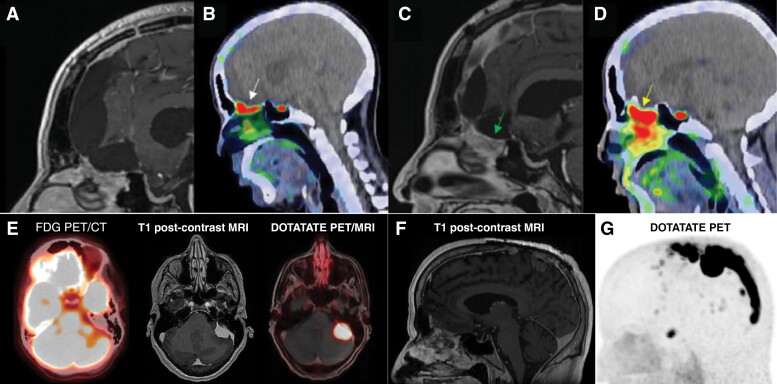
(A) Postoperative magnetic resonance imaging suggesting a gross total resection with contrast-enhancing reactive changes only. (B) Positron emission tomography (PET) imaging showing focal uptake along the cribriform plate (standardized uptake value 7.43, white arrow) suspicious for residual disease. (C) Follow-up MRI 2 years later after patient declined to pursue recommended adjuvant RT with increased enhancing soft tissue signal (arrow). (D) Increased focal PET uptake in the cribriform plate suggests progression of residual disease (standardized uptake value 8.96, yellow arrow). (E) Axial brain MRI of a different patient: a 54-year-old woman with newly diagnosed breast cancer metastatic to axillary lymph nodes who was noted to have asymmetric photopenia in the left cerebellum on a staging fluorodeoxyglucose (FDG)-position emission tomography (PET) and computer tomography (CT) scan (left). T1 post-contrast brain magnetic resonance imaging (MRI) showed a multilobulated, homogeneously enhancing extra-axial mass adjacent to the left petrous temporal bone with associated edema and mass effect in the left cerebellum and cerebellar peduncle (middle). Leading differential diagnoses included a distant metastasis or a meningioma. DOTATATE PET/MRI showed markedly avid uptake in the intracranial mass (right), but not in the right breast or ipsilateral lymph nodes (not shown). A diagnosis of synchronous meningioma and locoregionally advanced breast cancer was made. The meningioma was treated with stereotactic radiosurgery (SRS). The patient underwent lumpectomy, sentinel lymph node biopsy, and adjuvant whole breast radiotherapy. At 24 months after meningioma treatment and 13 months after breast cancer treatment, the patient had no evidence of disease. (F) Sagittal T1 post-contrast brain MRI (left) and DOTATATE PET (right) of a 61-year-old male with recurrent atypical meningioma, CNS WHO grade 2, status post resection and stereotactic radiosurgery 8 years before developing multiple vertex recurrences that were treated with subtotal resection. Planning DOTATATE PET imaging revealed extensive tumor infiltration of the sagittal sinus from the vertex to the torcula. Part of this figure was originally published in The International Journal of Radiation Oncology, Biology, Physics. Prasad et al. ^68^Ga-DOTATATE PET: The Future of Meningioma Treatment (2022).^152^ FDG, fluorodeoxyglucose; MRI, magnetic resonance imaging; PET, positron emission tomography; used with permission.
